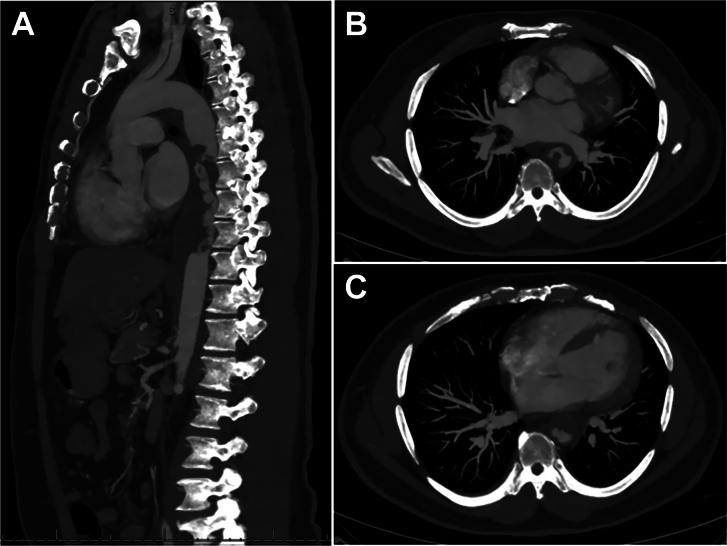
(A) Computed tomography angiogram (CTA) in sagittal plane. (B) Axial CTA. (C) Axial CTA.
